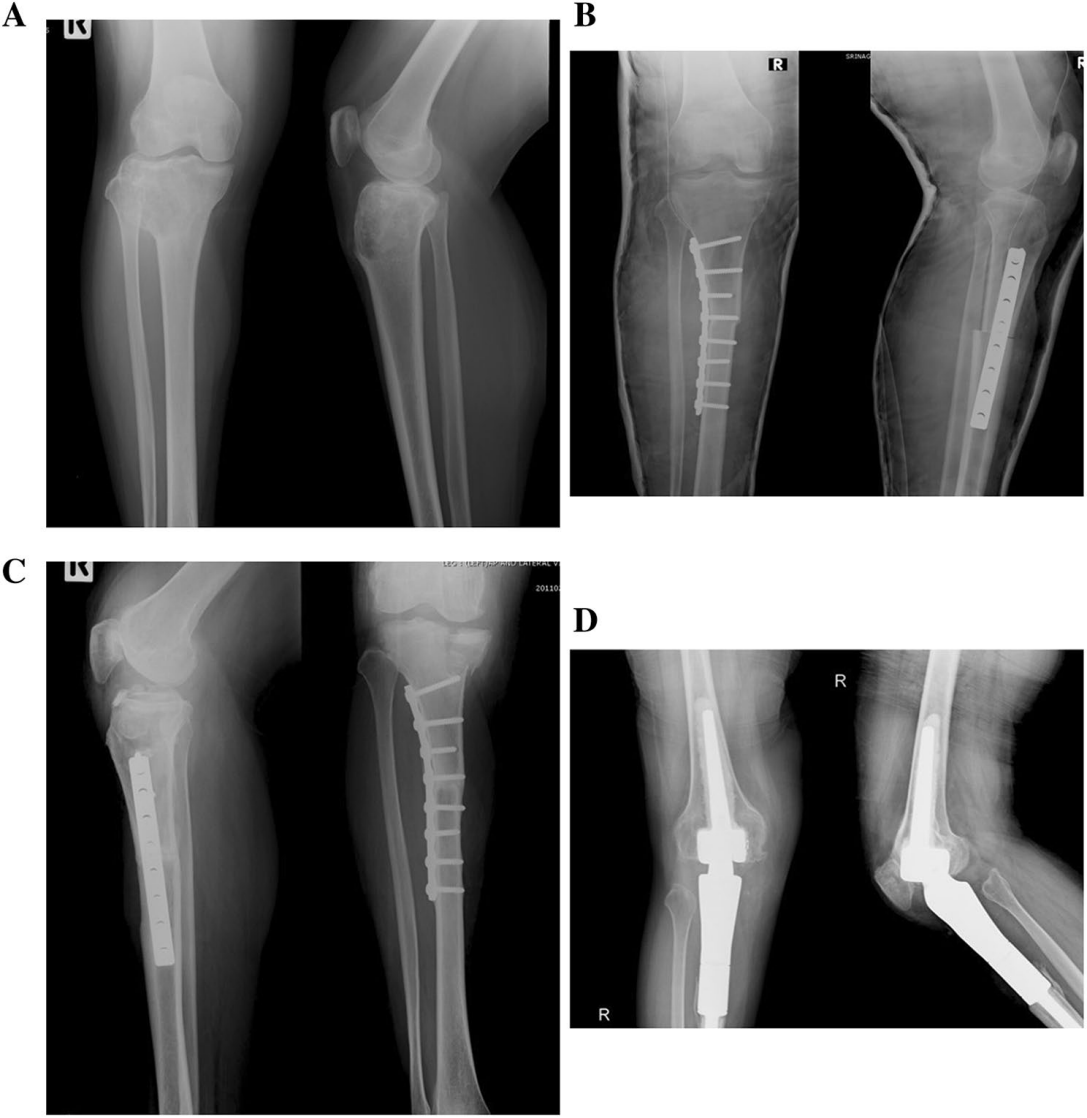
A 33-year-old female with osteosarcoma of the right proximal tibia. (A) Pre-operative anteroposterior and lateral radiograph. (B) After adjuvant chemotherapy, wide resection and reconstruction with proximal tibia allograft were performed. (C) Fracture of allograft (medial tibial condyle) occurred 5 years after reconstruction. (D) Revision surgery with proximal tibia endoprosthesis successfully performed.
